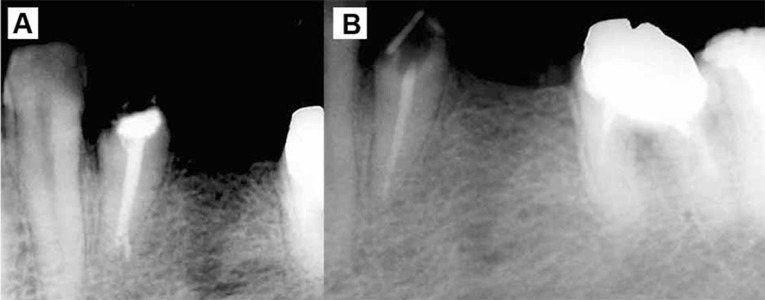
A) Radiographic image of the tooth #21 before retreatment; B) Radiographic image of tooth #21 after retreatment 11 years before taking CBCT image
